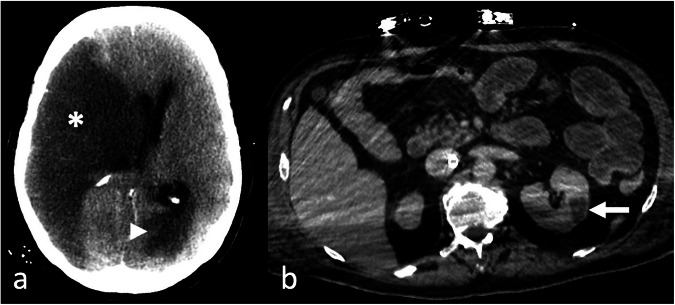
Unenhanced head computed tomography (CT) (a) in a 55-year-old patient on extracorporeal membrane oxygenation (ECMO) therapy, shows an extensive ischemic area in the right middle cerebral artery (MCA) territory (asterisk) and a smaller ischemic area located in the left parieto-occipital region (arrowhead), territory of the left posterior cerebral artery (PCA); increased attenuation represents a pseudo-subarachnoid hemorrhage, secondary to engorgement of venous structures due to increased intracranial pressure. Axial CT image in the venous phase (b) of a 65-year-old male with femoro-atrial veno-venous (VV)-ECMO reveals a hypoattenuating area in the left kidney (arrow), indicating ischemic damage
